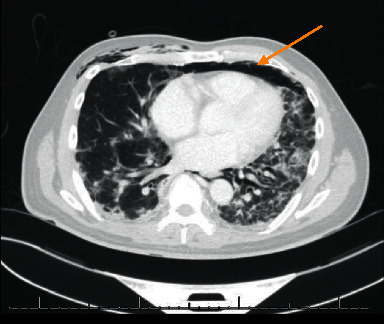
Lung CT scan demonstrating SPM (arrow) prior to lung transplant.
